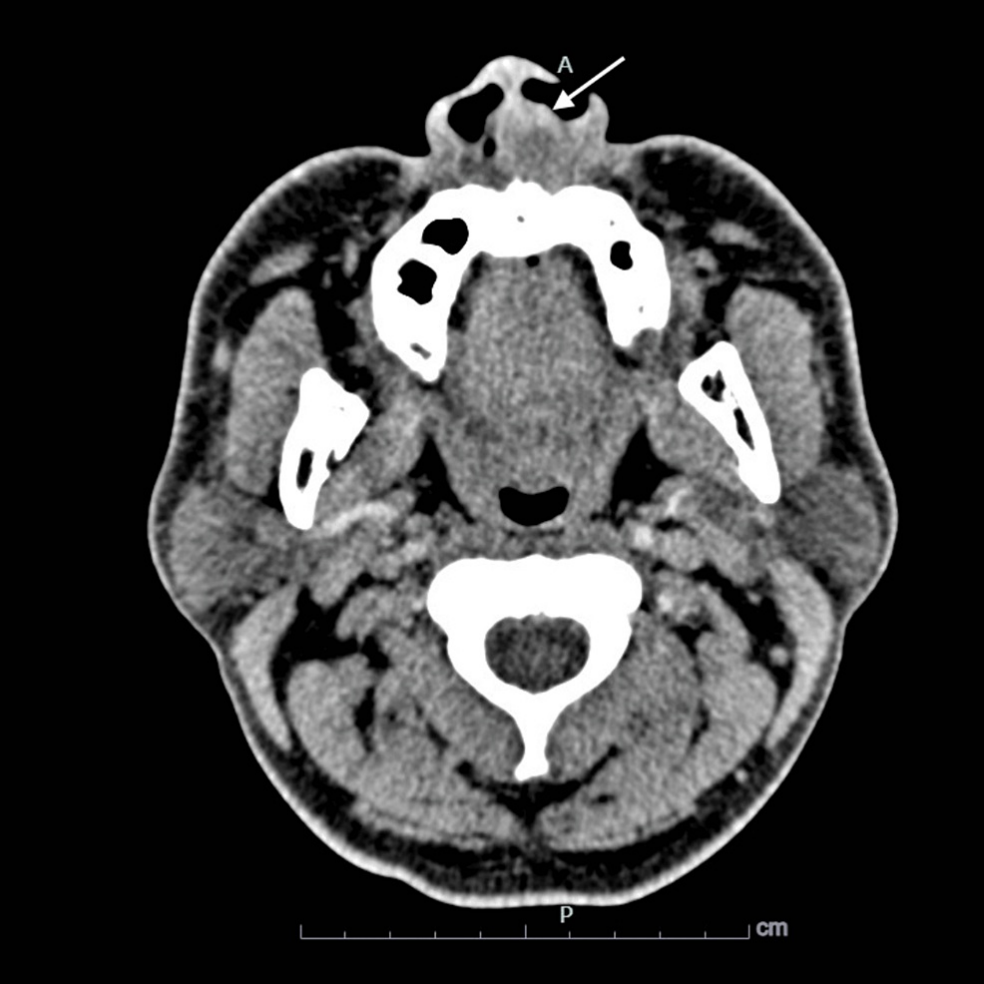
Computed tomography scan of the paranasal sinuses and post-nasal space, showing a lesion with a thickened, enhancing wall and a hypodense area at the anterior aspect of the nasal septum. This lesion, featuring erosion and right deviation of the nasal septum, is consistent with an early-forming abscess.
